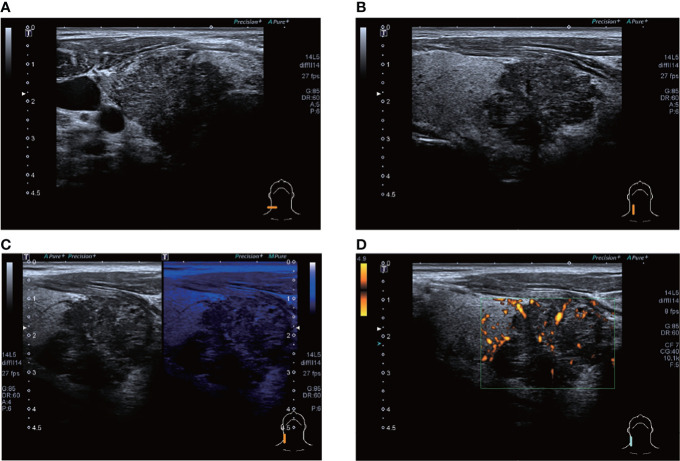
ultrasound images of patient #4 showing a hypoechoic nodule, which appears to be lobulated, in the lower pole of the right lobe of the thyroid gland, with punctate calcifications visible within. CDFI shows a grade 1 blood flow signal. The patient’s postoperative pathology was that of a highly differentiated squamous carcinoma, which has not recurred to date. (A) Lateral scan (B) Sagittal scan (C) "Firefly" technique (D) PDI.
